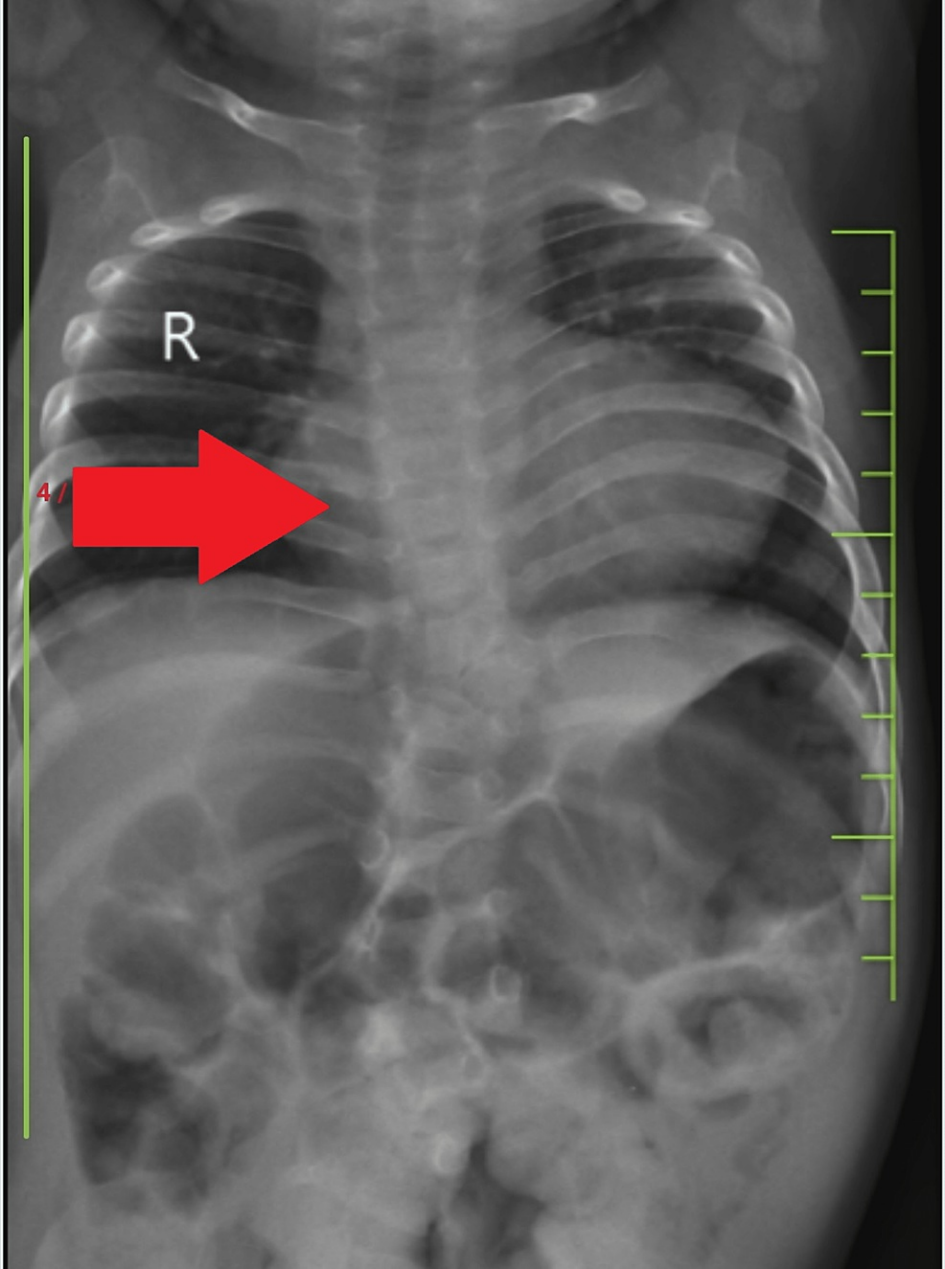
X-Ray Chest posteroanterior view suggesting boot-shaped heart
